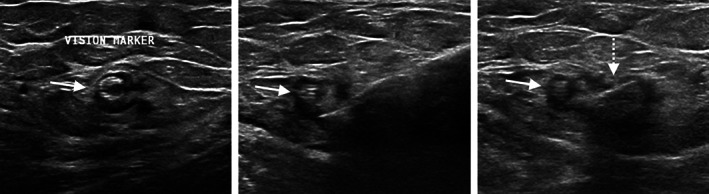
Ultrasound images during SmartClip™ localization of the tagged left axillary lymph node containing a Tumark Vision™ marker (solid arrow). (A). The applicator is placed into the lymph node with the bevel adjacent to the marker, (B), and the Purple SmartClip™ is deployed (dashed arrow), C
